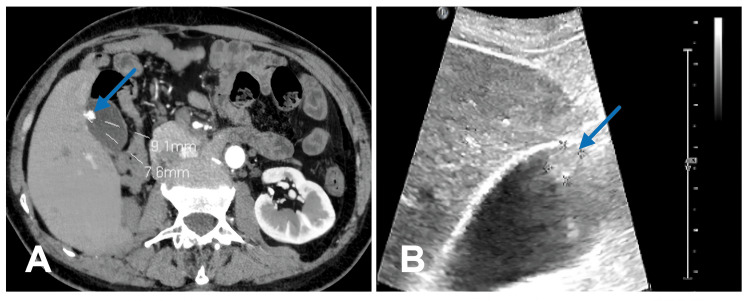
CT and ultrasound images of the patient A: CT image showing a nodule on the gallbladder wall, which demonstrates significant enhancement in the enhanced scan with a size of 9.1 mm × 7.6 mm; B: Ultrasound image showing a high echo protrusion on the gallbladder wall with multiple punctate strong echoes
